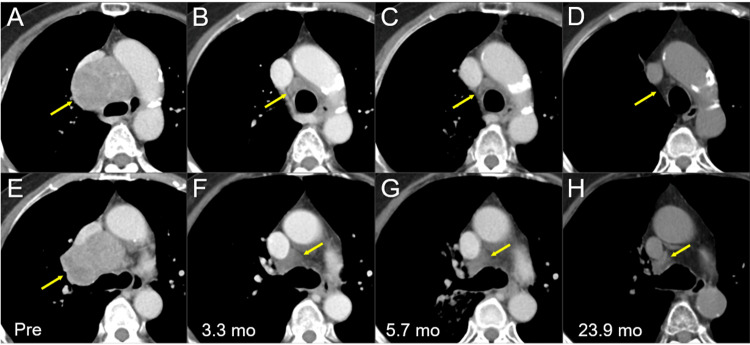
Thoracic computed tomography images before and after chemoradiotherapy. The images show axial (A-C, E-G) CE CT images and axial (D, H) non-CE-CT images (A, E) 28 days before (Pre) the initiation of CRT, (B, F) at 3.3 mos after CRT initiation (after four courses of EC), (C, G) at 5.7 mos (after six courses of EC), and (D, H) at 23.9 mos. (A-H) These images are shown at the same magnification and to some extent the same coordinates under co-registration and partly manual fusions. (A, B) Well-demarcated heterogeneously enhanced mass lesions (arrows in A and E) in the mediastinum and right hilum, with the displacement of the trachea, bilateral bronchi, esophagus, and superior vena cava. (B, C, F, and G) The lesions regressed remarkably, however, still leaving the residual masses (arrows in B, C, F, and G). (D, H)  The residual mass lesions further regressed (arrows in D and H) at 23.9 mos. CE, contrast-enhanced; CT, computed tomography, CRT, chemoradiotherapy; mo, month; EC, etoposide plus cisplatin
